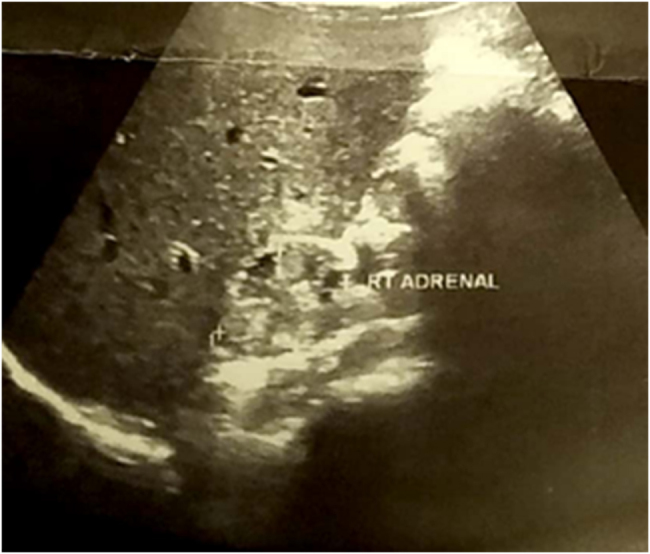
Abdominal ultrasound shows a hyperechoic, heterogeneous, 3.5-cm right adrenal mass.
